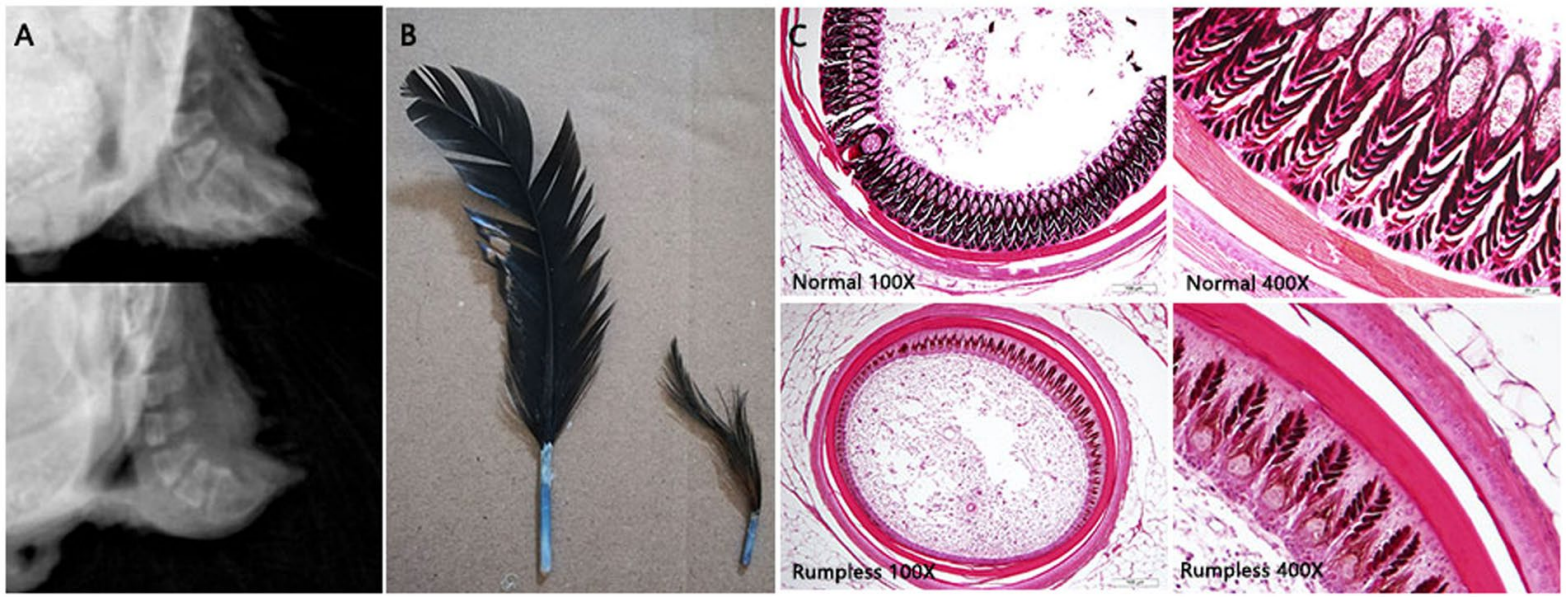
Morphological observations of two types of Hongshan chicken. (A) X-ray image of normal (top) and rumpless (bottom) rooster. (B) A picture of the main tail feather of normal (left) and rumpless (right) roosters. (C) Section of a feather in the feather follicle at 100 × (left) and 400 × magnification (right); normal rooster on top and rumpless rooster at the bottom.
